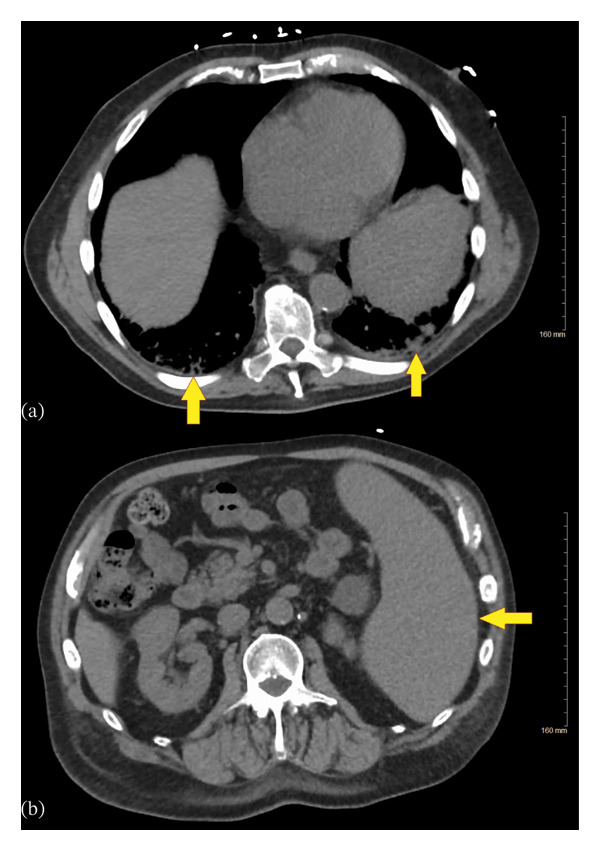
Computed tomography of the chest and abdomen in our patient on initial presentation. (a) Axial section at lower thoracic level demonstrating bibasilar infiltrates and atelectasis (arrows). (b) Axial section at upper abdominal level demonstrating splenomegaly (arrow).
